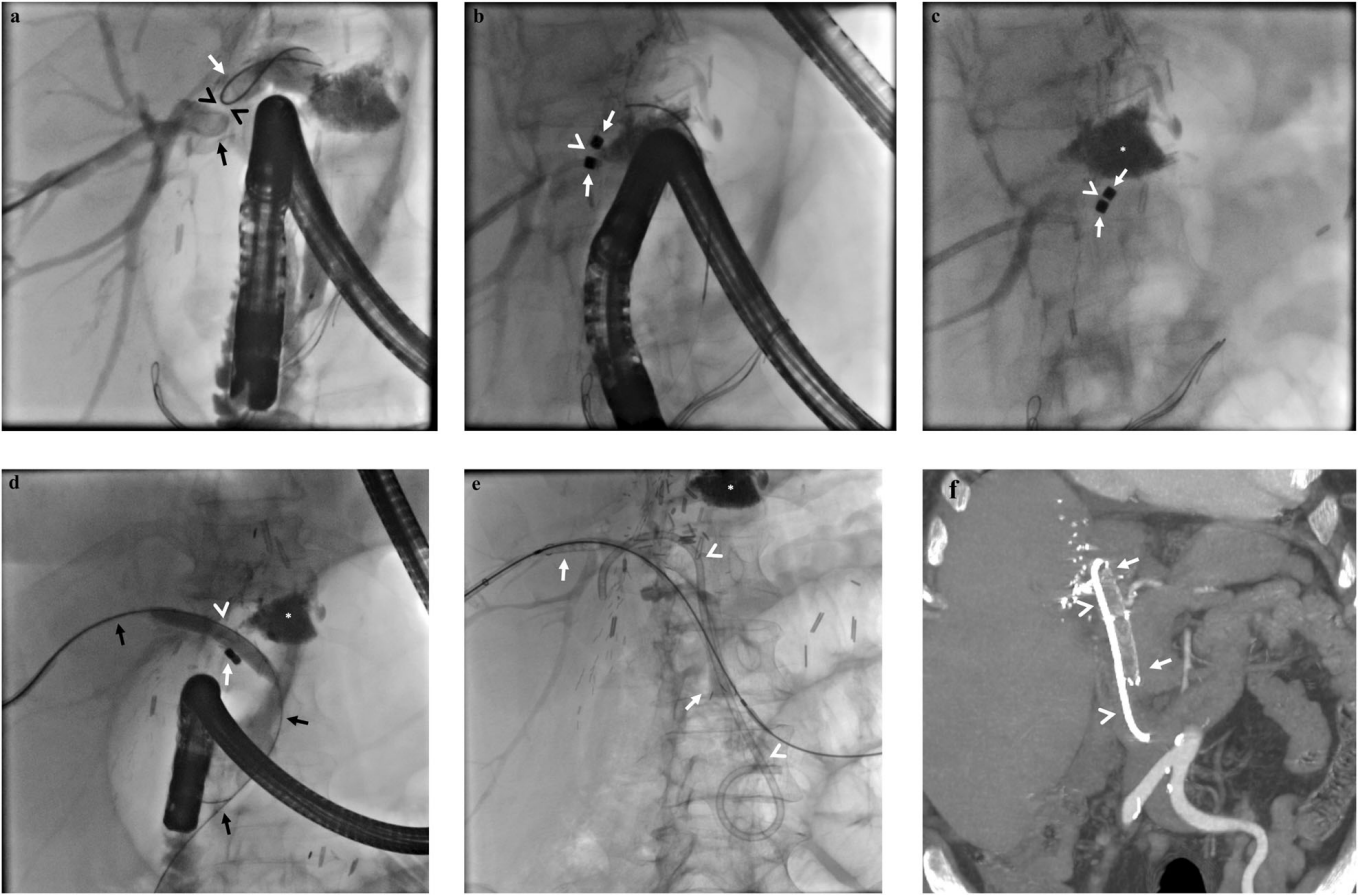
A 67-year-old woman developed biliary stricture 8 months after receiving living donor liver transplantation due to hepatitis B cirrhosis. a Cholangiography demonstrates complete biliary obstruction at duct-to-duct anastomosis site. Note intervening soft tissue thickness (arrowheads, a) between two wires. Both cranial (percutaneous approach, black arrow, a) and caudal (ERCP, white arrow, a) wire accesses had failed in traversing the obstruction, therefore magnetic compression anastomosis was decided. b, c Two magnets were pushed over the wires simultaneously via a transhepatic and ERCP approach (arrows, b, c). Immediate approach of the magnets was noted. The gap between the magnets (arrowheads, b, c) was reduced due to magnetic adherence. d Following complete apposition of the magnets, wire access beyond the obstruction was able to be established on the 4th day following magnet placement (black arrows, d). Magnets (white arrow, d) were pushed down to the duodenum with the support of a balloon catheter (arrowhead, d). e Finally, a fully covered metallic stent (arrows, e) along with a PS (arrowheads, e) was placed, and the percutaneous biliary drain was removed. Note metallic stent is not yet fully expanded due to stricture. f Coronal contrast-enhanced CT scan obtained 5 months later due to epigastric pain, revealed a fully expanded metallic stent (arrows, f) and PS (arrowheads, f). No sign of biliary dilatation is also noted. An asterisk indicates changes related to the previous vertebroplasty procedure
